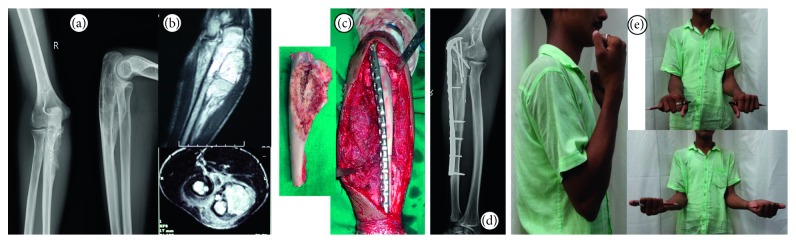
16-year-old male diagnosed with Ewing's sarcoma of the right proximal ulna presented after receiving neoadjuvant chemotherapy with radiographs (a) and MRI (b). He underwent an intercalary through the olecranon resection and reconstruction (c) with extracorporeal irradiation of resected segment. At latest follow-up of 42 months, there is no evidence of joint arthritis (d) or disease recurrence. Both osteotomy junctions have healed, and patient has excellent function (e).
